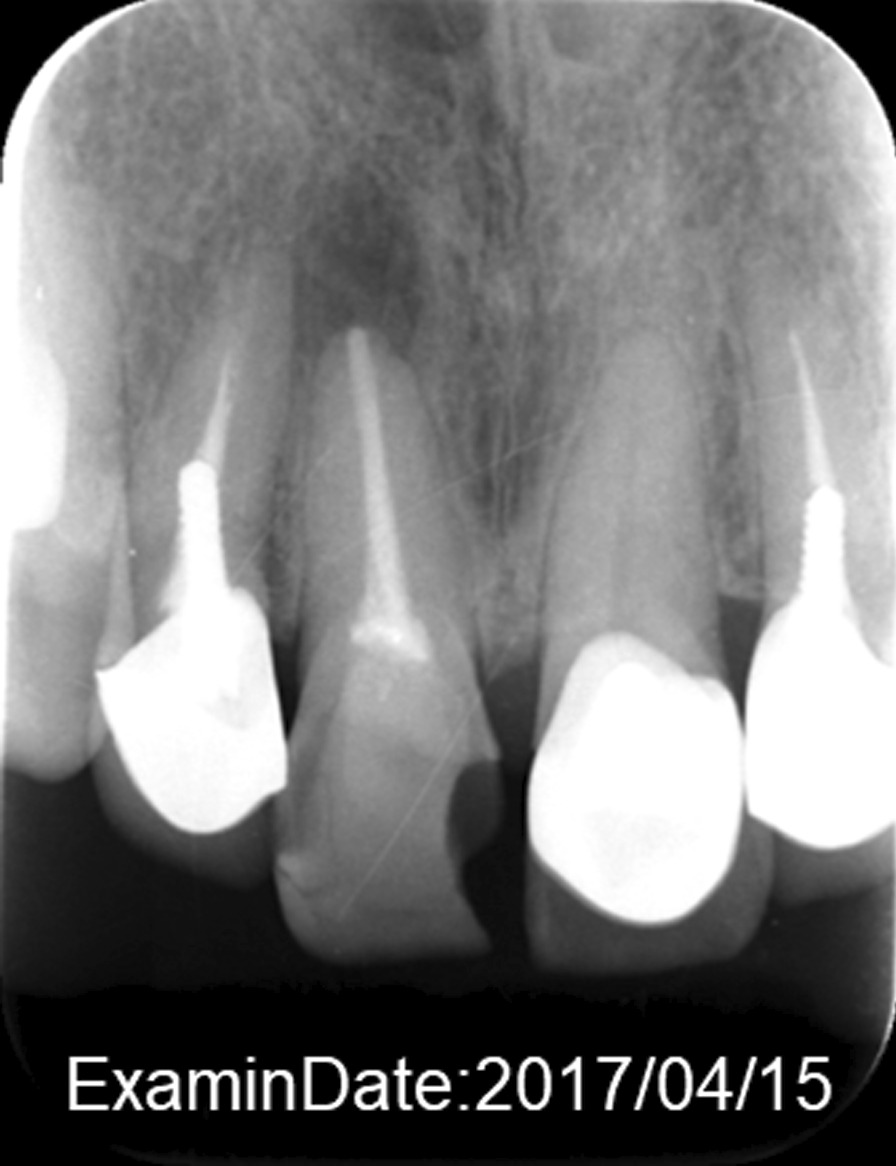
Radiograph of the upper front tooth on 15 April 2017 during the first dental examination. This image shows that the front tooth is missing
